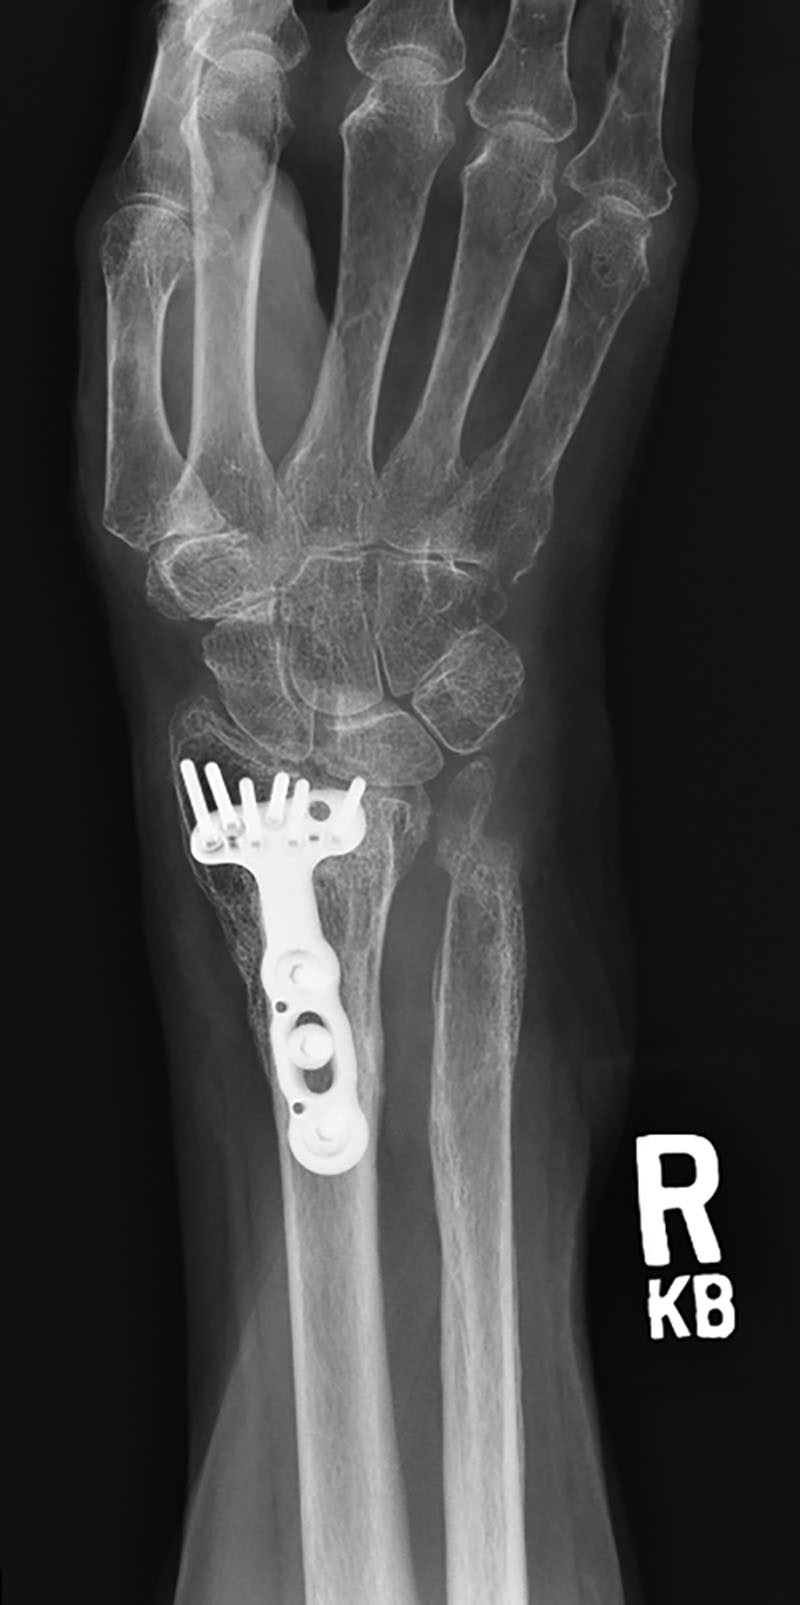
Patient 2 8-month postoperative roentgenogram showing no recurrence of heterotopic ossification.
